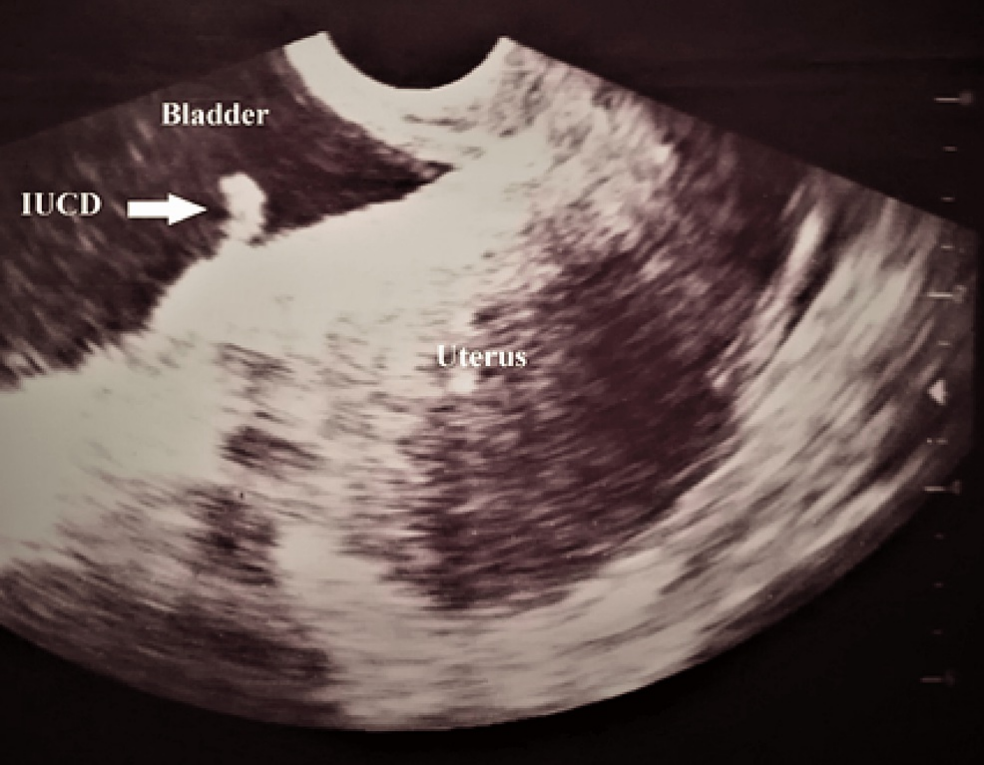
TVS with full bladder showing the IUCD (arrow) within the bladder cavity jutting from the posterior wall of the bladder TVS: transvaginal ultrasound; IUCD: intrauterine contraceptive device
